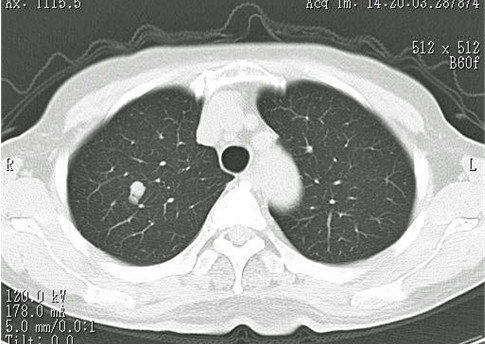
Chest CT showing the tumor, 10 mm in diameter, in S1.
